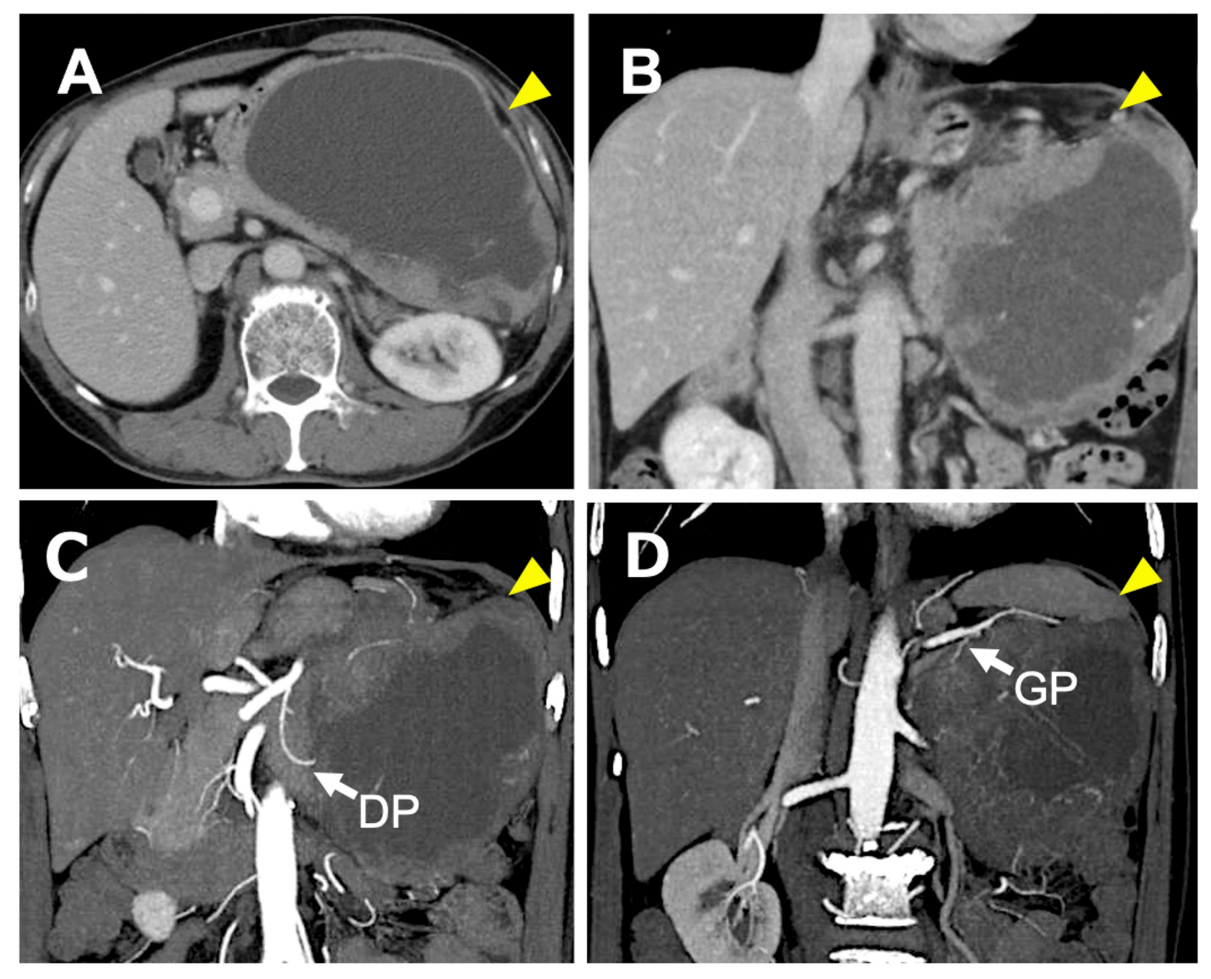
Contrast-enhanced computed tomography (CT) of the abdomen. (A) Axial and (B) coronal images demonstrate a giant heterogeneous tumor measuring approximately 15 cm in maximum diameter, arising in the left upper abdominal cavity. The tumor exhibits internal cystic degeneration consistent with necrosis and prominent peripheral enhancement (arrowheads).
